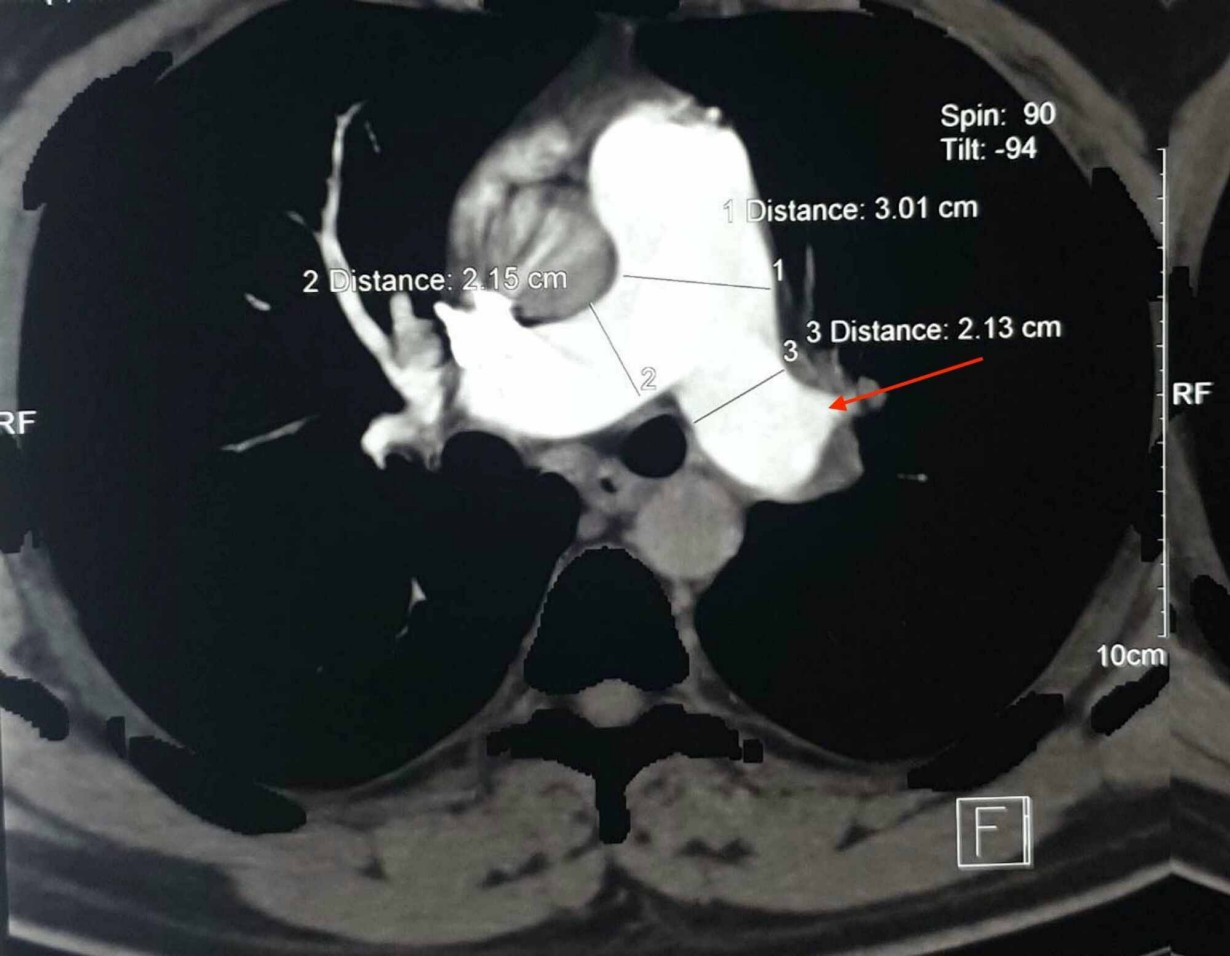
Axial section of CTPA scan showing reduction in the size of the filling defect in left main pulmonary artery. CTPA - Computed tomography pulmonary angiogram
